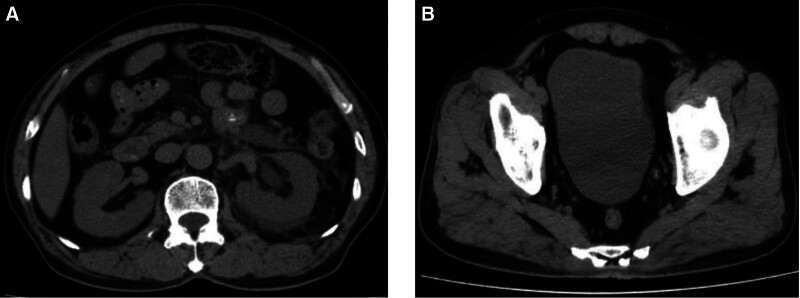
Abdominal computed tomography. (A) Exudative changes in the left kidney. (B) Sediment calculi changes in the left lower ureter.
